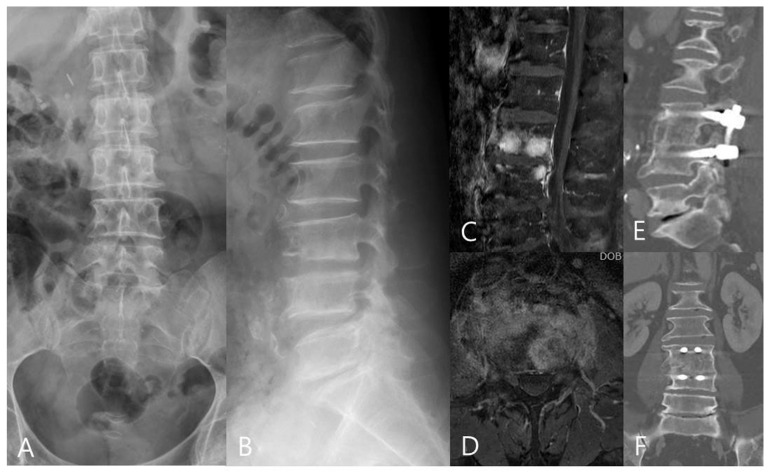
A 53−year−old woman had lower back pain for more than 2 months. (A,B) The preoperative AP, lateral radiographs, and (C,D) MRI images revealed L3/4 pyogenic spondylodiscitis with endplate destruction. An anterior lumbar interbody fusion with a tri-cortical iliac bone graft was performed. (E,F) CT images at the last follow-up showed L3/4 interbody fusion and good sagittal alignment.
